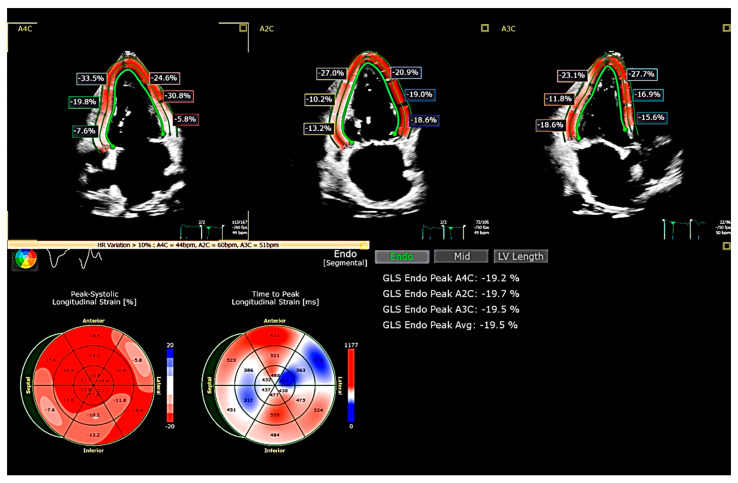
LV GLS (assessed using speckle-tracking echocardiography) of a patient affected by dilated cardiomyopathy with borderline low ejection fraction (LVEF 51%) but significantly impaired longitudinal contraction of basal septum, basal lateral, and mid-basal inferior walls. GLS = Global Longitudinal Strain; LV = Left Ventricle; LVEF = Left Ventricular Ejection Fraction.
